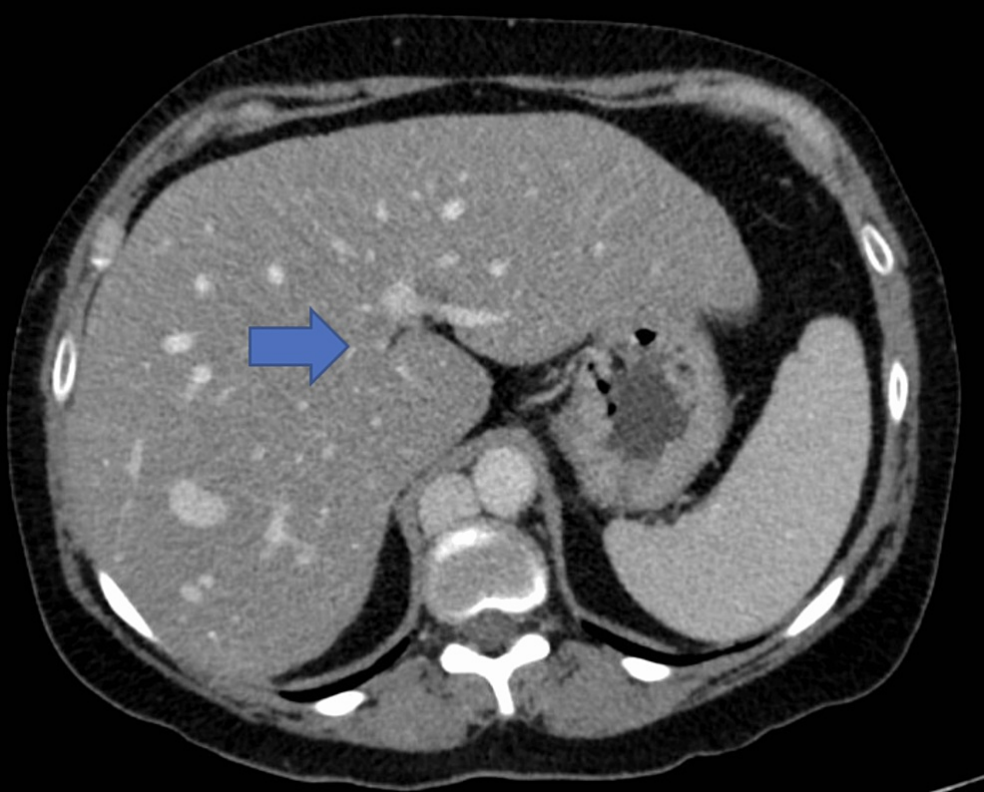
CT abdomen and pelvis, axial reconstruction, reveals the complete absence of the intrahepatic part of the inferior vena cava (blue arrow)
